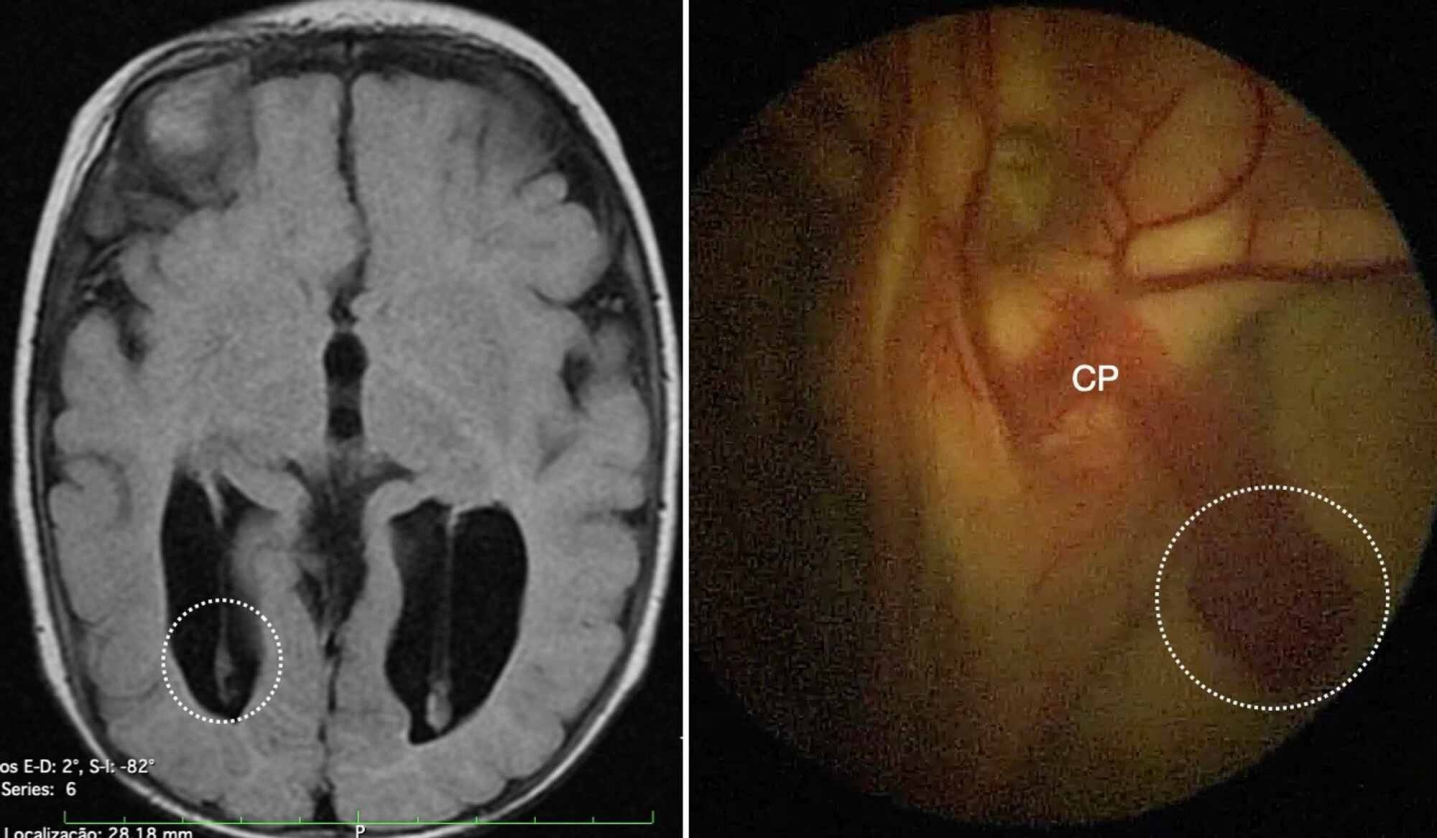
The type III anatomic variation of the glomus of choroid plexus (GCP). Type III GCP is classified as mobile, and the elongation is extreme. On both sides, the GCP (indicated by the white dotted circle) is closer to the posterior limit of the occipital horn, as observed in an axial view on T1-weighted magnetic resonance imaging (left). In this endoscopic view of the right lateral ventricle, the GCP is mobile and suitable for resection by our technique. CP, choroid plexus.
